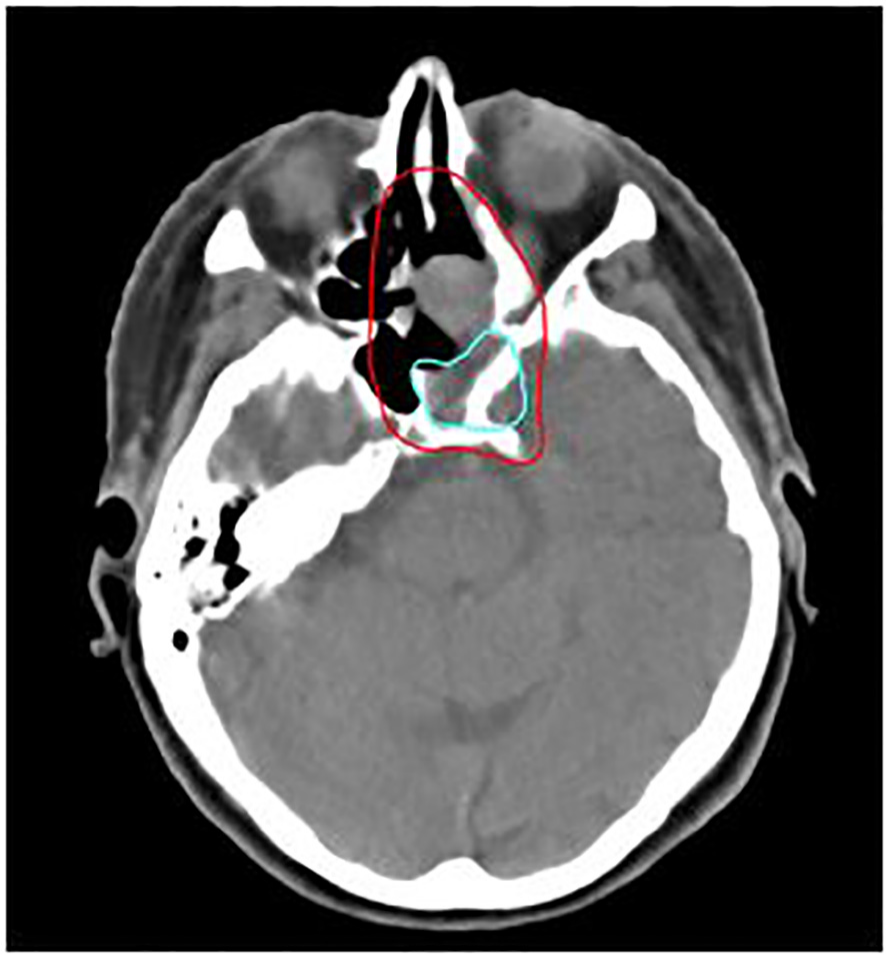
76-year-old female patient with mucosal melanoma of the paranasal sinus treated with bimodal intensity-modulated radiotherapy (IMRT) in combination with carbon ion therapy. The patient developed a local relapse (light blue) located in-field of the C12 boost CTV (red), delineated on the planning CT.
